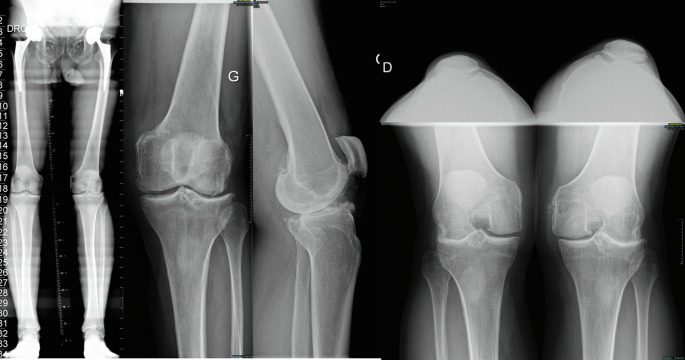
Preoperative x-rays of a valgus knee with osteoarthritis treated with image-based total knee arthroplasty following the principles of functional knee positioning, using a lateral approach. The case is presented in Video 1.
